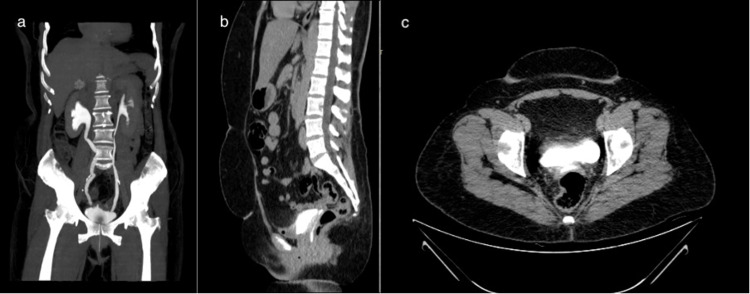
Multiplanar CT evaluation of the abdomen and pelvis: coronal, sagittal, and axial views. (a) Coronal reconstruction showing right moderate hydronephrosis. (b) Sagittal section (delayed phase) of CECT demonstrating contrast leak from bladder into vaginal canal. (c) Cross section (delayed phase) of CECT demonstrating contrast leak from bladder into vaginal canal.
